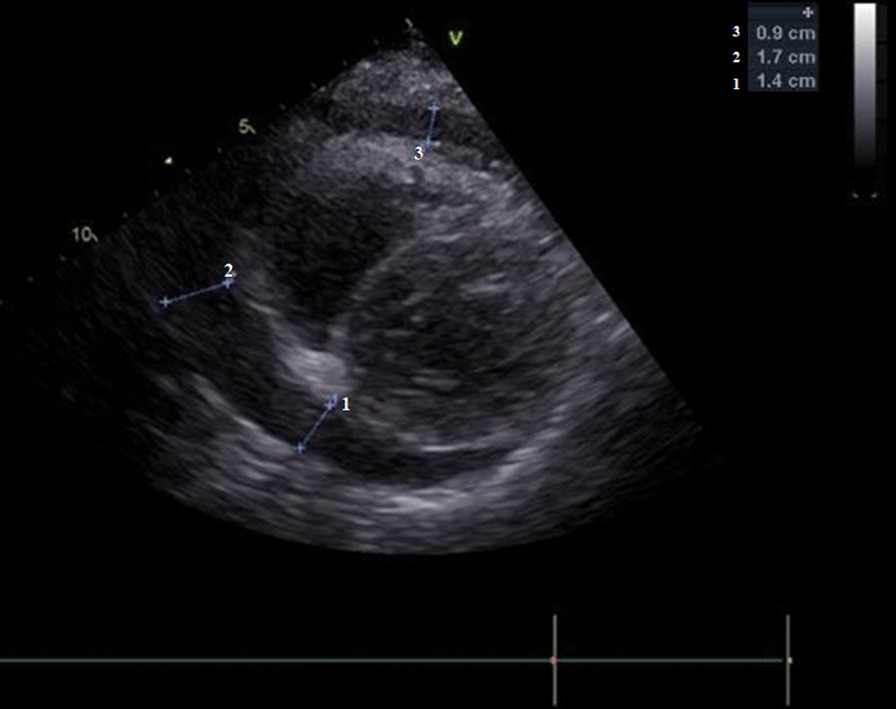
Echocardiographic imaging revealing the presence of significant pericardial collection
